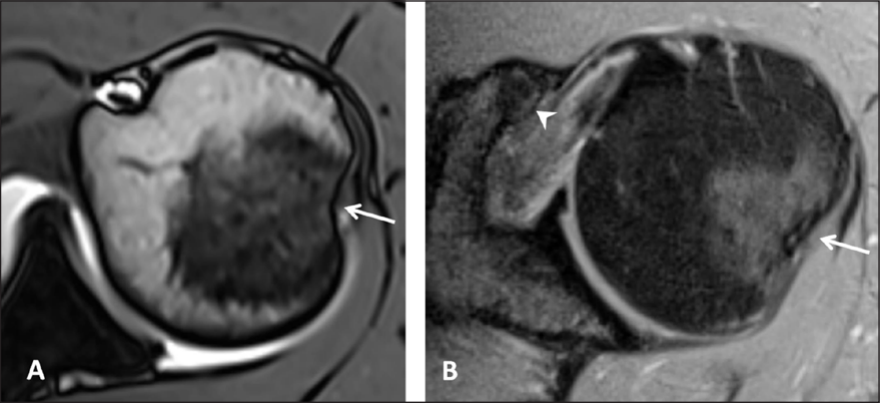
Normal humeral head versus Hill-Sachs lesion. (A) On the axial T2 gradient echo weighted MR image, there is a slight flattening of the posteroinferior surface of the humeral head (arrow), which is a normal finding. (B) Axial fat saturated T2-weighted MR image obtained at the level of the coracoid process (arrowhead, B) typically shows a Hill-Sachs defect (arrow) in a patient with history of anterior shoulder dislocation.
